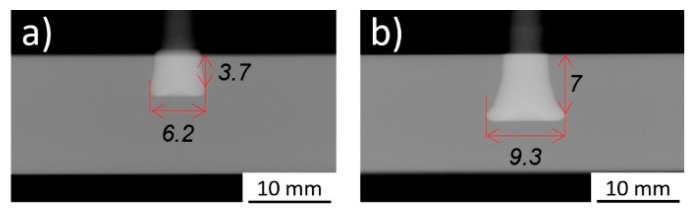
X-ray tomography of: (a) Condition 1 (E_M_ = 24 J; RS = 18,000 rpm; FT = 1.6 s; FoT = 1 s; FF = 2000 N; FoF = 5100 N); (b) Condition 4 (E_M_ = 77 J; RS = 20,000 rpm; FT = 2 s; FoT = 1 s; FF = 2000 N; FoF = 5100 N).
